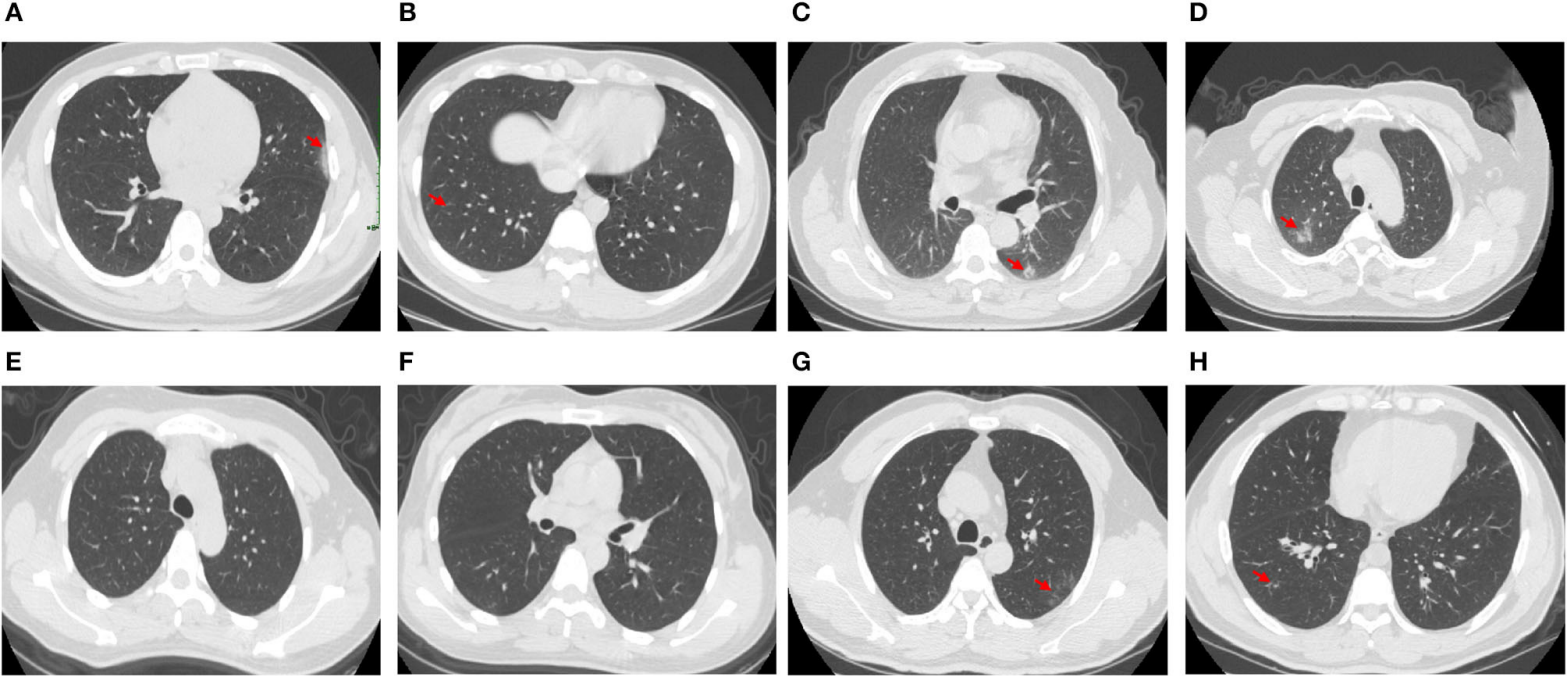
CT images of 4 patients whose N protein is consistently negative, (A,B), CT images of Patient 3 1–3 days after admission; (C,D), CT images of Patient 32 1–3 days after admission; (E,F), CT images of Patient 33 1–3 days after admission; (G,H), CT images of Patient 40 1–3 days after admission.
